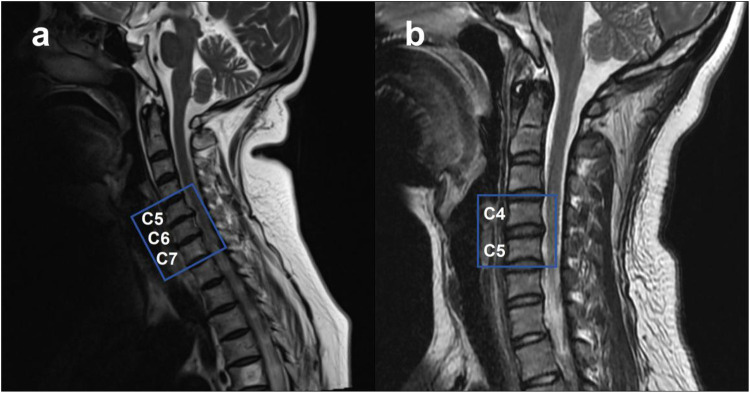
MRI of the cervical spine. (a) Cervical spine MRI of Patient 1 demonstrates loss of physiological lordosis, anterior osteophyte formation at the C5–C6 and C6–C7 levels, and disc herniation. (b) Cervical spine MRI of Patient 2 shows cervical lordosis and a mild C4–C5 disc herniation.
